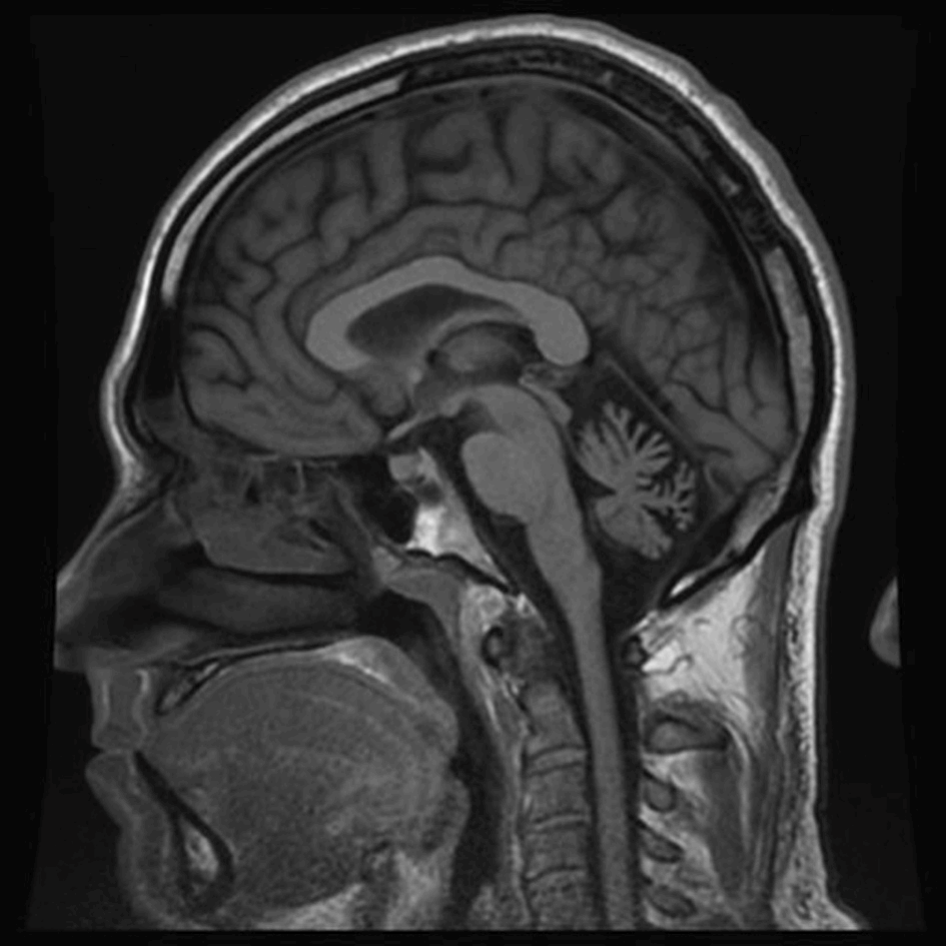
MRI examination (T1 weighted sagittal image) of patient F. Notice severe cerebellar atrophy.
